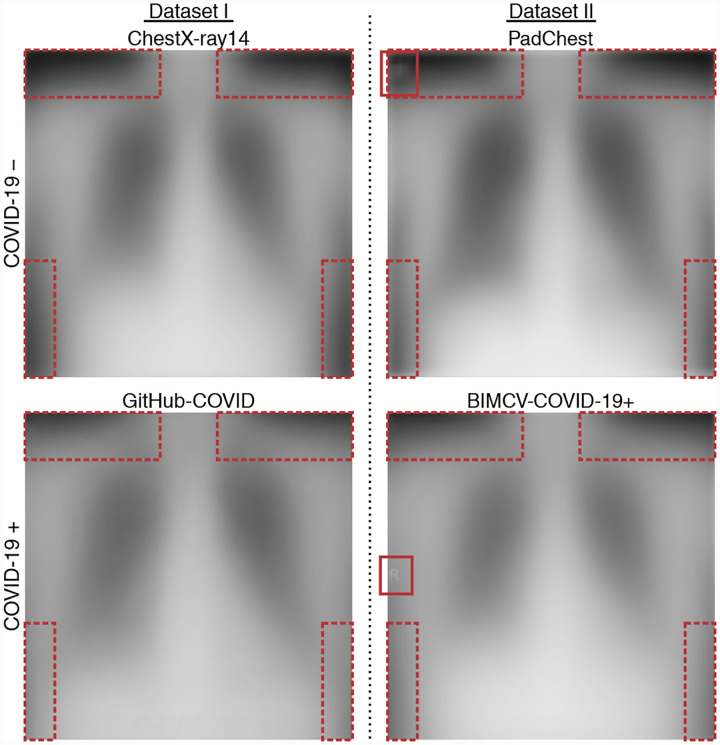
Average images from the four repositories used to construct datasets in this study, demonstrating systematic differences between the radiograph repositories that could be exploited by AI systems. Solid red boxes indicate systematic differences in laterality markers that are visible in the average images, and dashed red boxes indicate systematic differences in radiopacity of the image borders, which could arise from variations in patient position, radiographic projection, or image processing.
